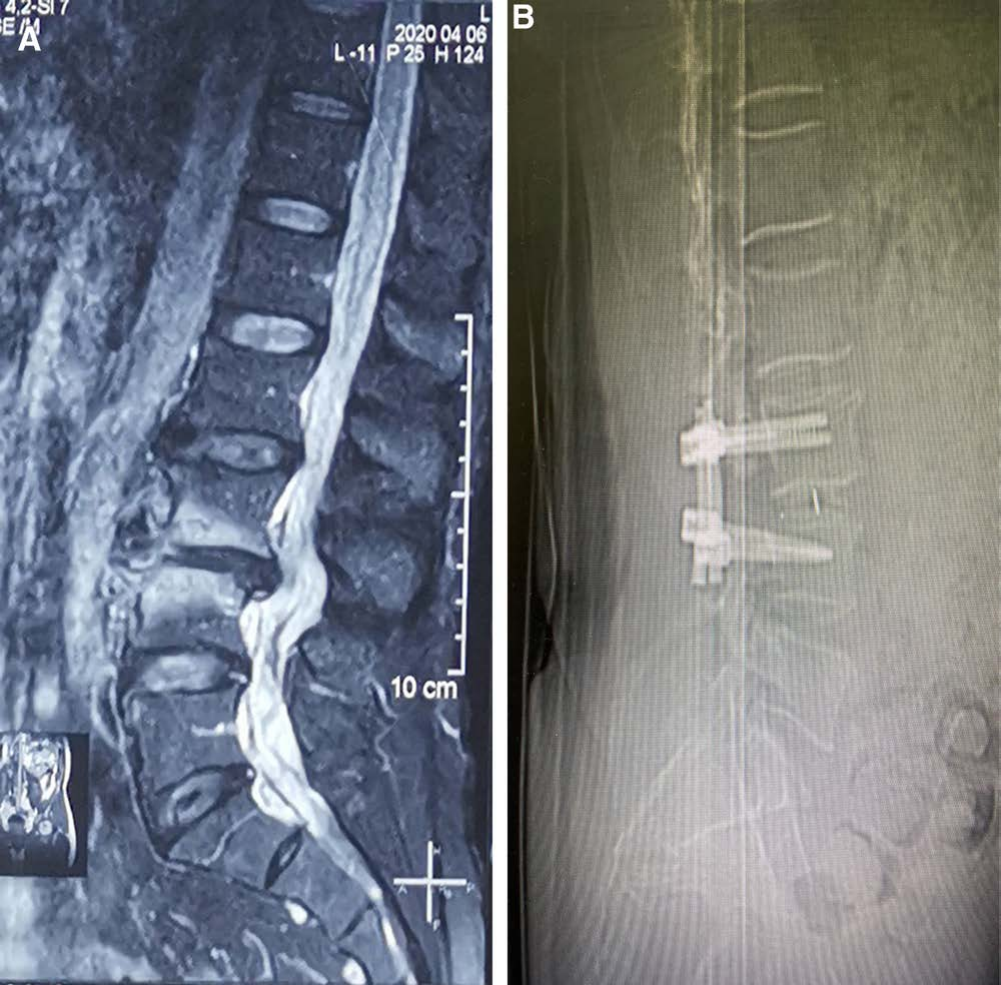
MR (A) before discectomy and fusion in the local hospital, and CT (B) after the operation in the local hospital.
